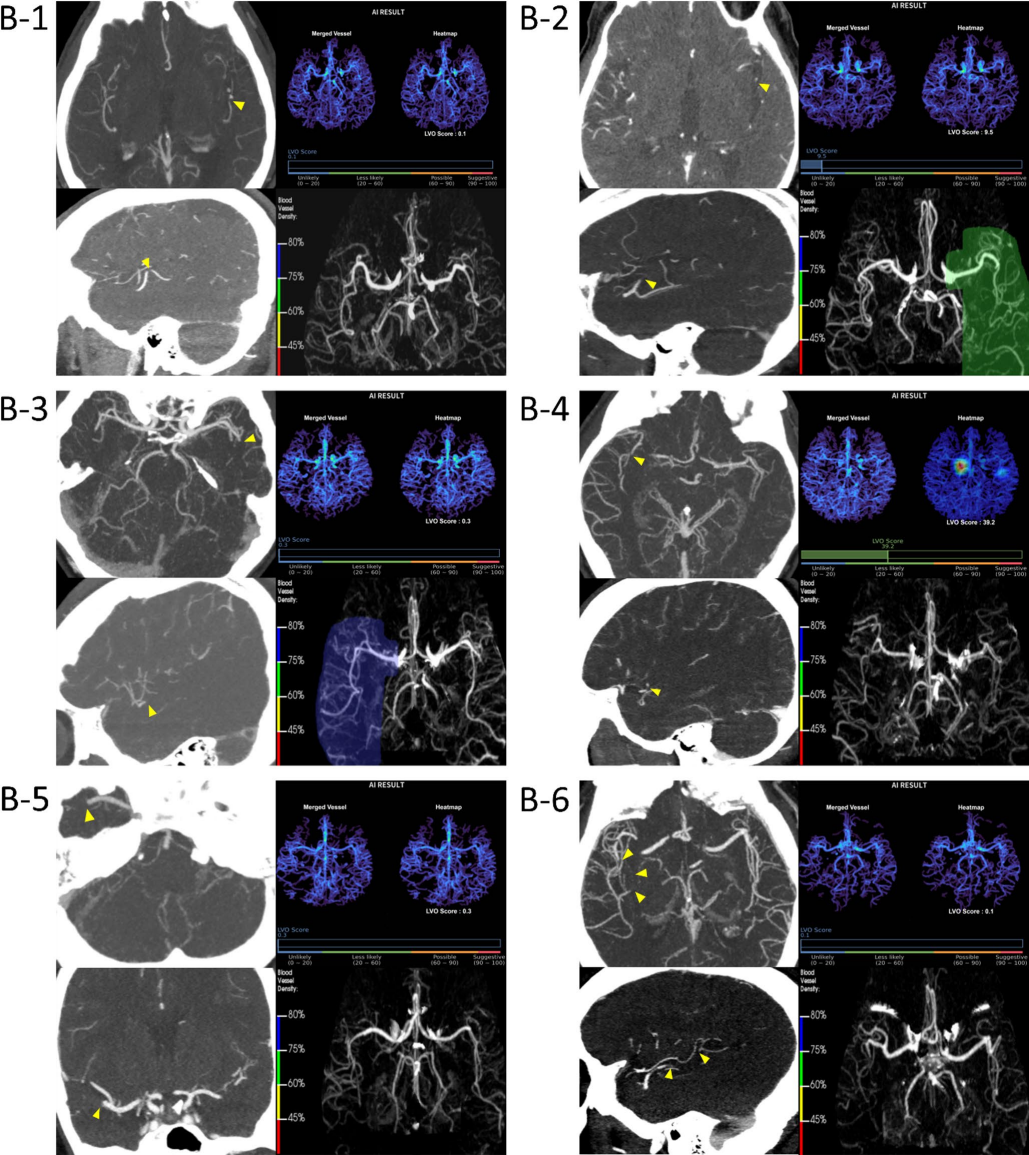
Common False-Positive and False-Negative Cases. (A-1) A 77-year-old male with focal moderate stenosis in the right MCA M1 segment (arrowheads), which was misinterpreted as an occlusion by both algorithms. (A-2) A 63-year-old male presented with a small amount of interpeduncular subarachnoid hemorrhage (arrowheads). There was no evidence of vasospasm or steno-occlusive disease. B-1) An 89-year-old male with a short-segment left MCA M2 occlusion (arrowheads). The finding was likely missed by both algorithms due to the short lesion length and good collateral flow. (B-2) A 71-year-old male with a left MCA M2 inferior division occlusion (arrowheads). The lesion was not detected, likely due to the preserved distal vessel density. (B-3) A 49-year-old male with an occlusion of a distal left MCA M2 branch (arrowheads). The false-negative result was likely due to the lesion's small caliber and the presence of moderate collateral circulation. (B-4) A 56-year-old male with a short-segmental occlusion of right MCA M2 inferior division (arrowheads) accompanied by focally poor collateral flow. The short lesion length likely contributed to the false-negative result. (B-5) A 26-year-old female with a right MCA M2 inferior division occlusion (arrowheads). The false-negative finding was likely attributable to robust collateral circulation. (B-6) An 89-year-old male with a segmental occlusion in the distal right MCA M2 inferior division (arrowheads), accompanied by good collateral flow. CTA= computed tomography angiography, MIP= maximum intensity projection, LVO= large vessel occlusion, MCA= middle cerebral artery
